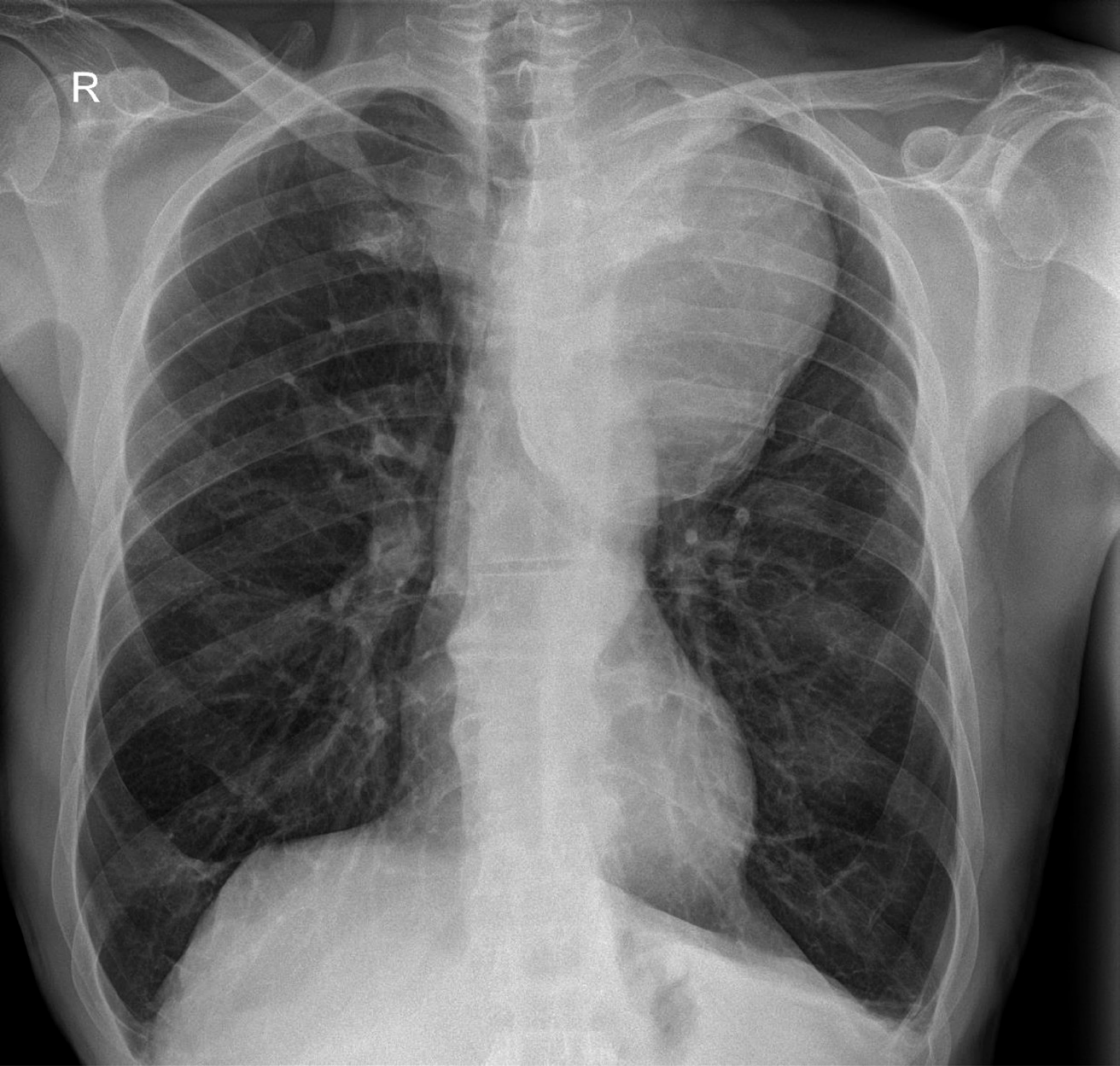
Pre-op chest X-ray with massive mediastinal mass with trachea deviated to the right
